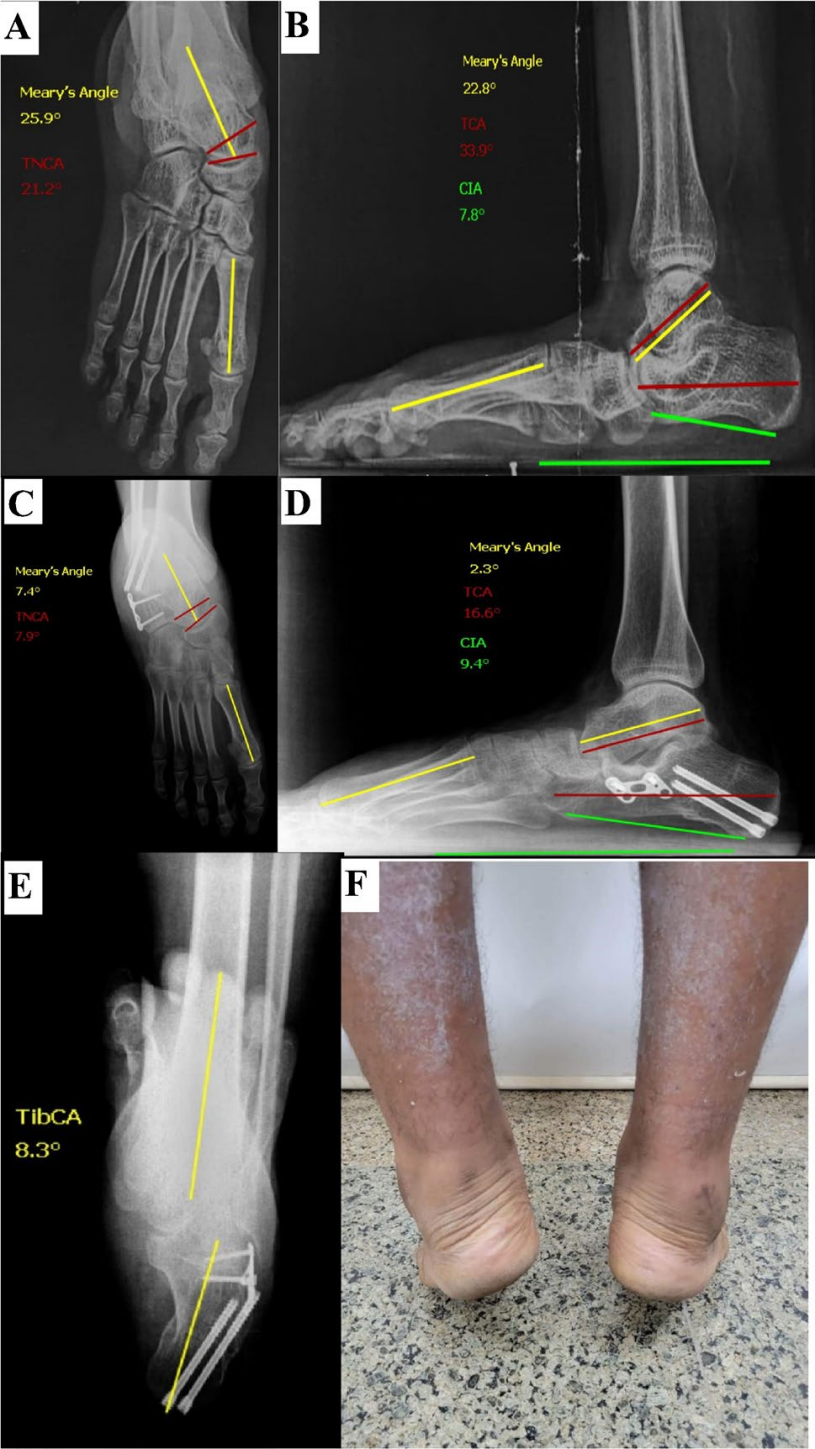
A male patient, 17 years old, had a DCO procedure. A, B Preoperative radiographs. C–E Last follow-up (20 months) radiographs showing deformity correction (as per angles measured) and restoration of the heel alignment as shown in the axial view. F A clinical image showing restoration of the heel alignment
